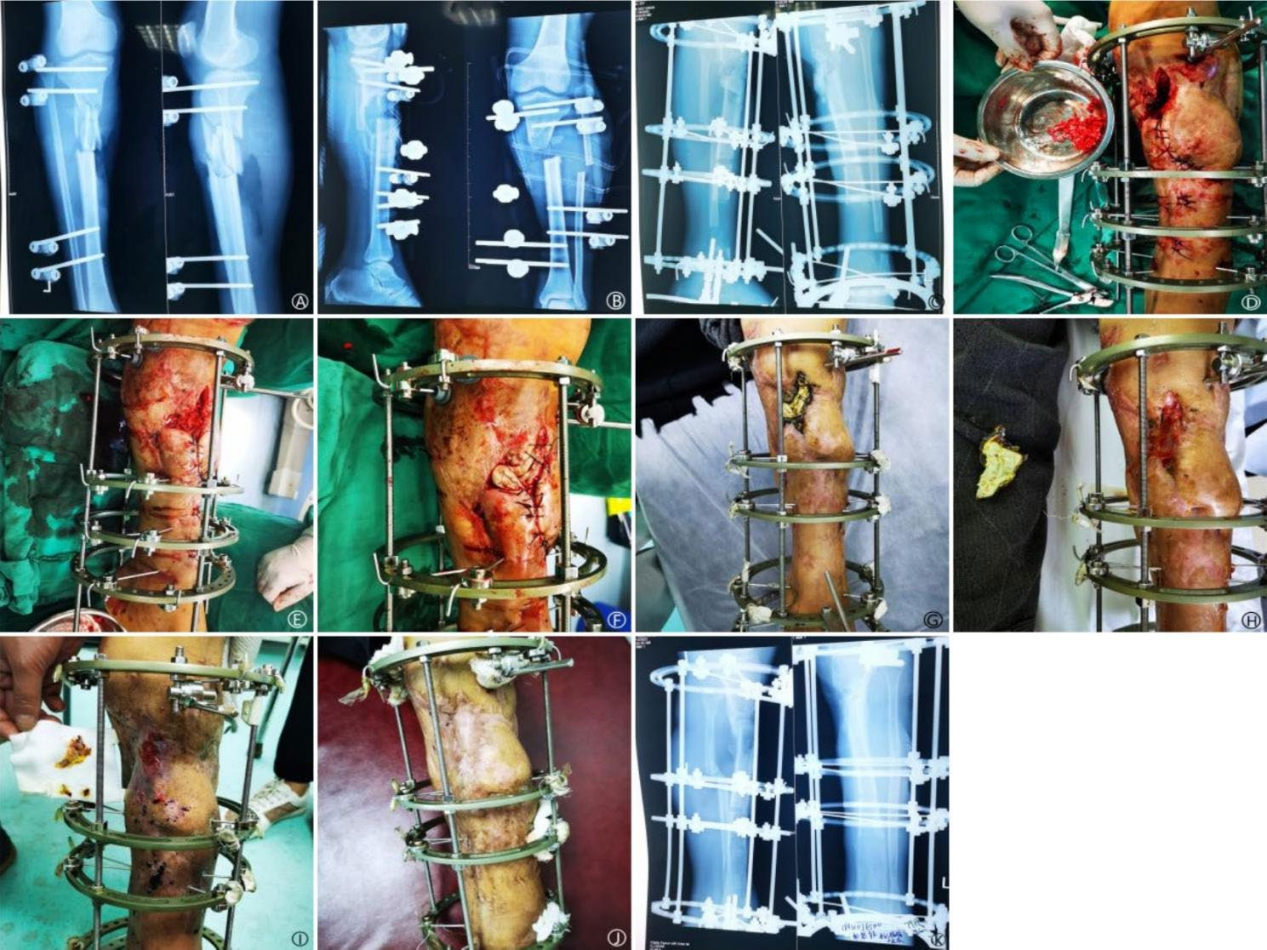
A: a 32-year-old man underwent open fracture of the left proximal tibia and wound infection after external fixation. B: after two debridements, the bone defect was 8 cm, with 2.5-cm distance between the amputated ends despite of shortening by 5.5 cm. C: X-ray at 2 months semi-open bone grafting and biplanar osteotomy. The proximal bone segment was moved 0.5 cm reversely to compress the bone graft end and the distal segment was lengthened by 6 cm to restore the length of the leg. D/E: preparation and implantation of cancellous bone graft granules from iliac bone mixed with 0.5-g vancomycin. F/G: coverage by bone cement sheet. H: fresh granulation tissue covered the bone graft at 2 months later. I/J: 4 and 6 months after bone grafting, respectively. K: X-ray at 7 months later
